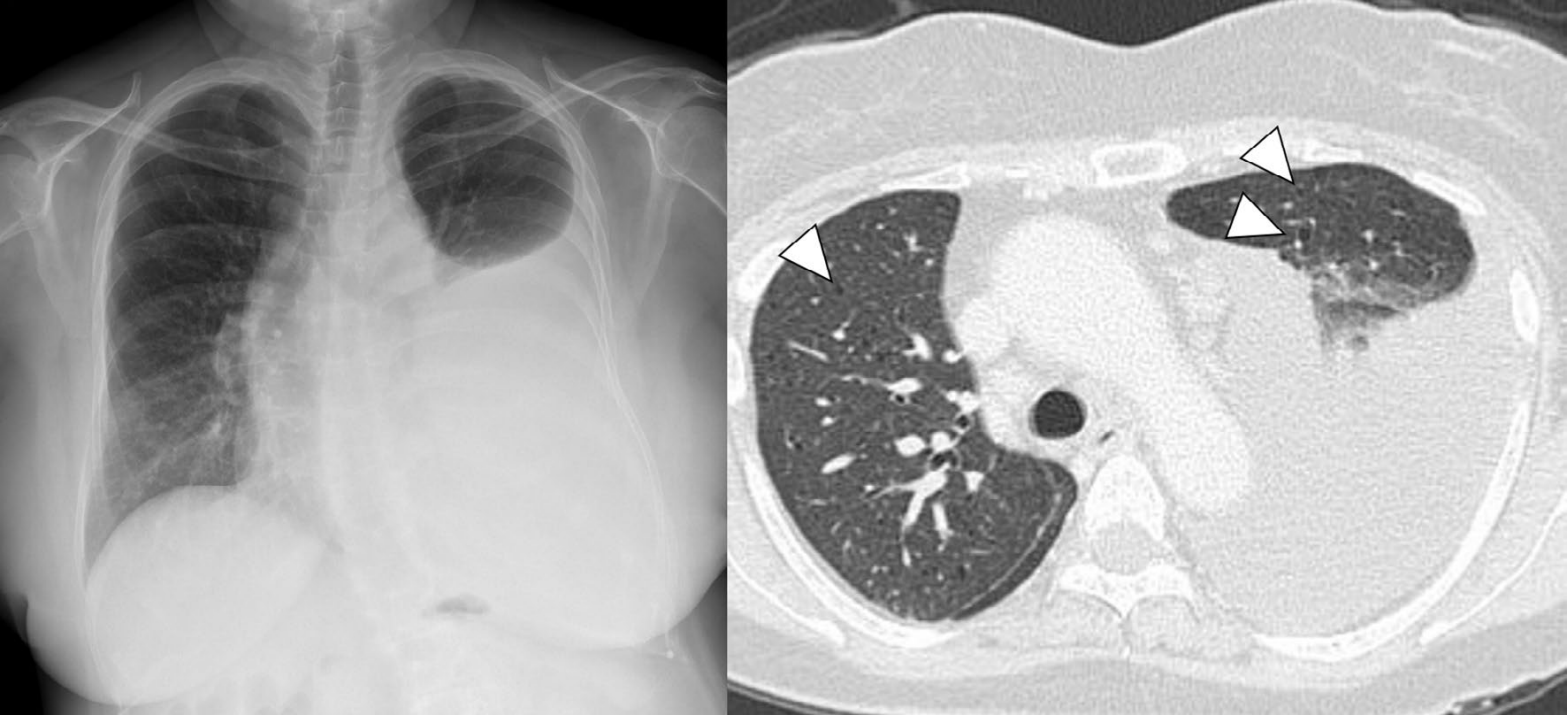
Chest radiograph revealed a left pleural effusion, and computed tomography revealed multiple thin‐walled cysts in both lung fields (white arrowheads).
